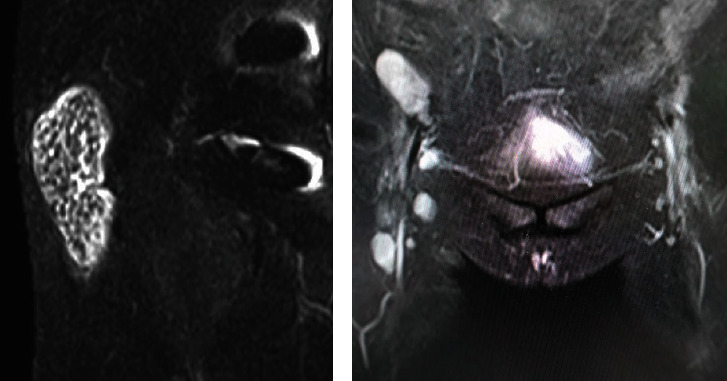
MRI. (a) Small honeycomb-shaped bodies within the cystic lesion on the T2-weighted STIR sequence. (b) Numerous inguinal lymphadenopathies on the T2-weighted STIR sequence.
